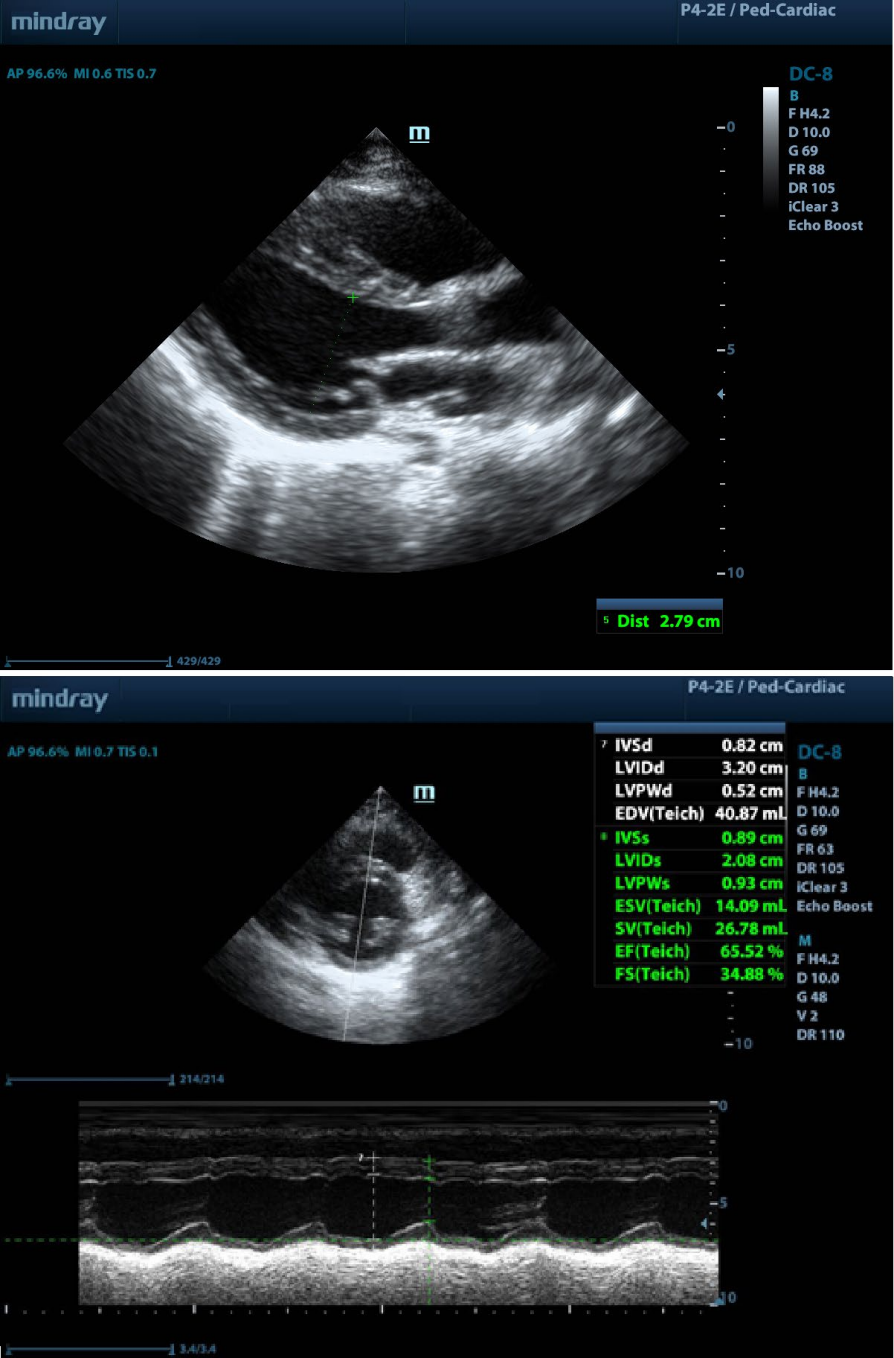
Case 2. The echocardiogram at 5 y of age with no heart chamber dilatation
